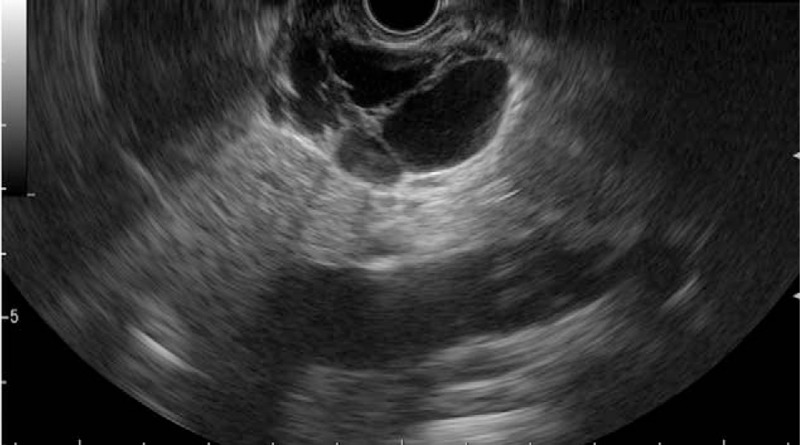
Endoscopic ultrasound findings. Multilocular cystic lesion along the pancreatic tail has thin multiple septum and mural nodules.
